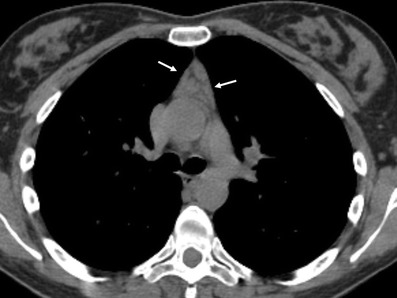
Thymic lymphoid hyperplasia in a 41-year-old woman with clinical diagnosis of myasthenia gravis. Non-contrast-enhanced CT scan shows an enlarged thymic gland (*arrows*) without mass effect on adjacent structures
